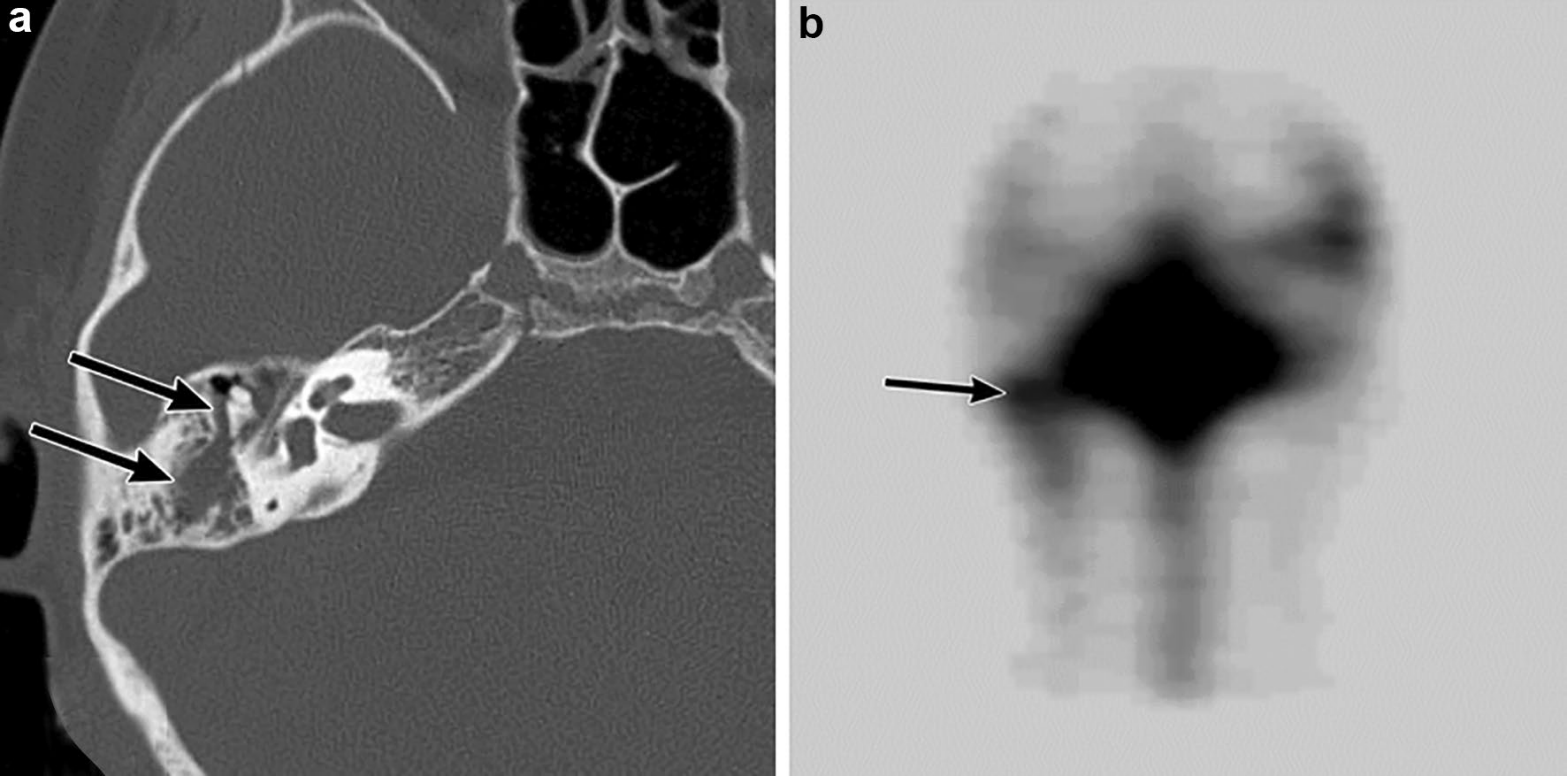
Cerebrospinal fluid (CSF) leak in the right middle ear in a 10-year-old boy who was diagnosed with bacterial meningitis five times. The patient had a head injury 8 years prior to presentation. a Axial temporal bone computed tomography shows fluid collection in the middle ear and mastoid air cells, suggestive of a CSF leak (black arrows). It is probably caused by remote head trauma, but fractures cannot be identified. b Radionuclide cisternography shows radionuclide accumulation in the right mastoid cells and the right middle ear, and a CSF leak was confirmed (black arrow). A lumbar-peritoneal shunt was performed to prevent the CSF leak. Surgical repair was not performed since the exact leak site remained unclear
